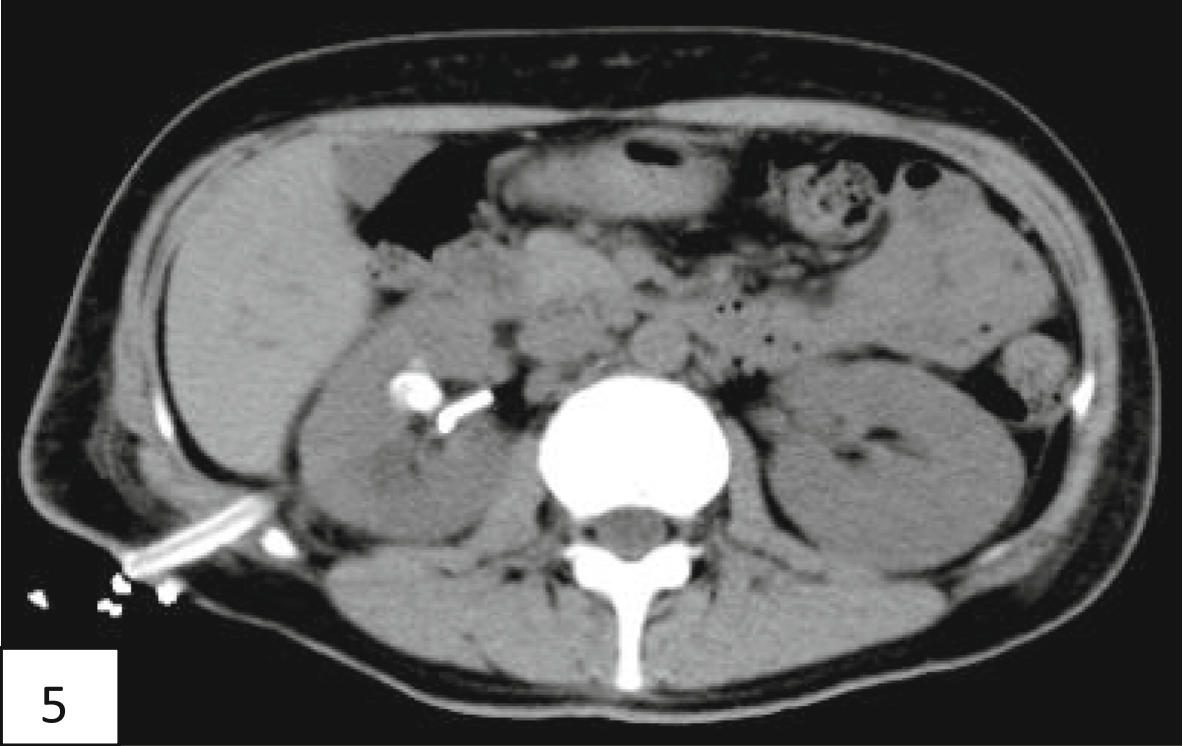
Shows the repeat non-contrast CT scan after the first percutaneous nephroscopy
